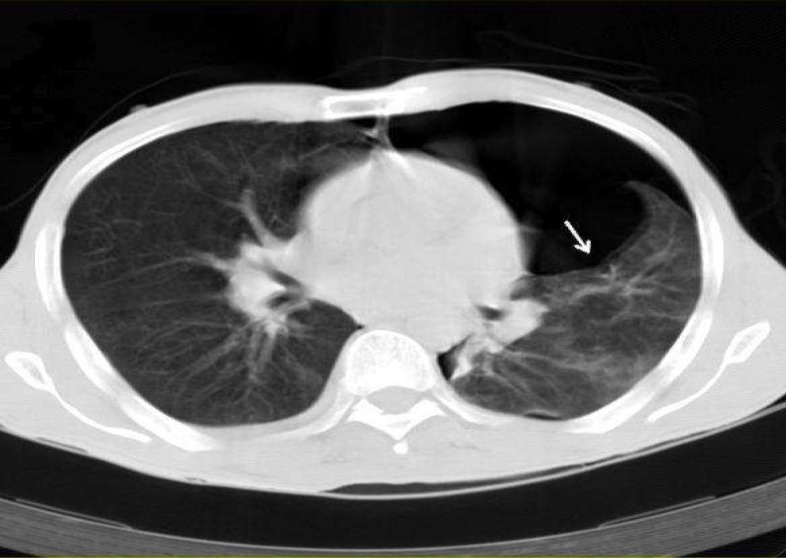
Chest CT scan uncovered a tension pneumothorax
